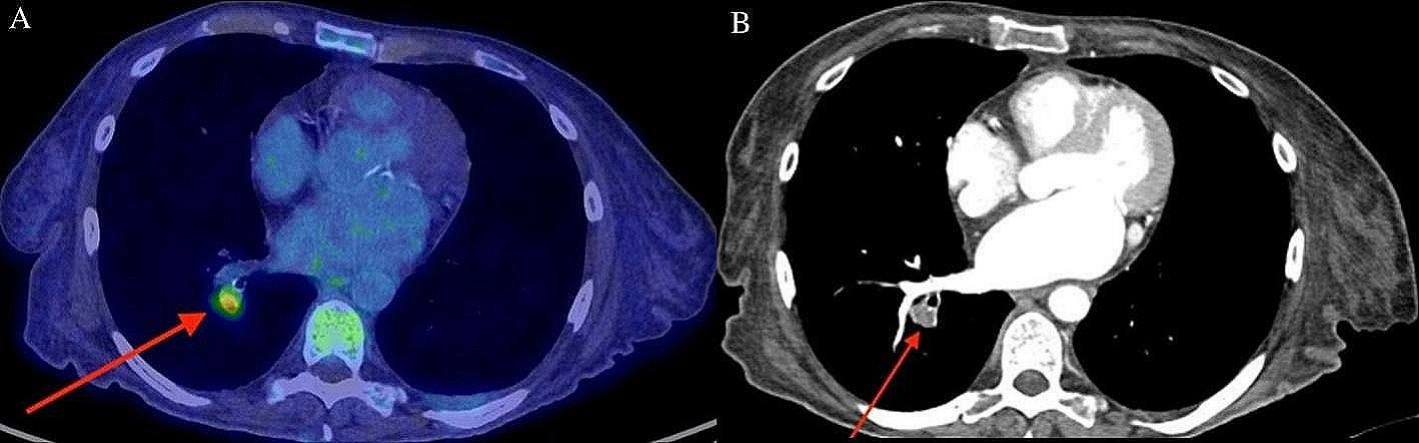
^18^F-FDG whole-body PET/CT and CT pulmonary angiography showed evidence of septic pulmonary embolus in RLL segmental arteries. ^18^F-FDG whole-body PET/CT revealed increased FDG uptake (SUVmax 3.8) in branching of segmental arteries from the right lower lobe (RLL) pulmonary artery, suspicious for septic pulmonary embolus (A, arrow). Subsequent CT pulmonary angiography study revealed RLL distal lobar and segmental emboli (B, arrow)
